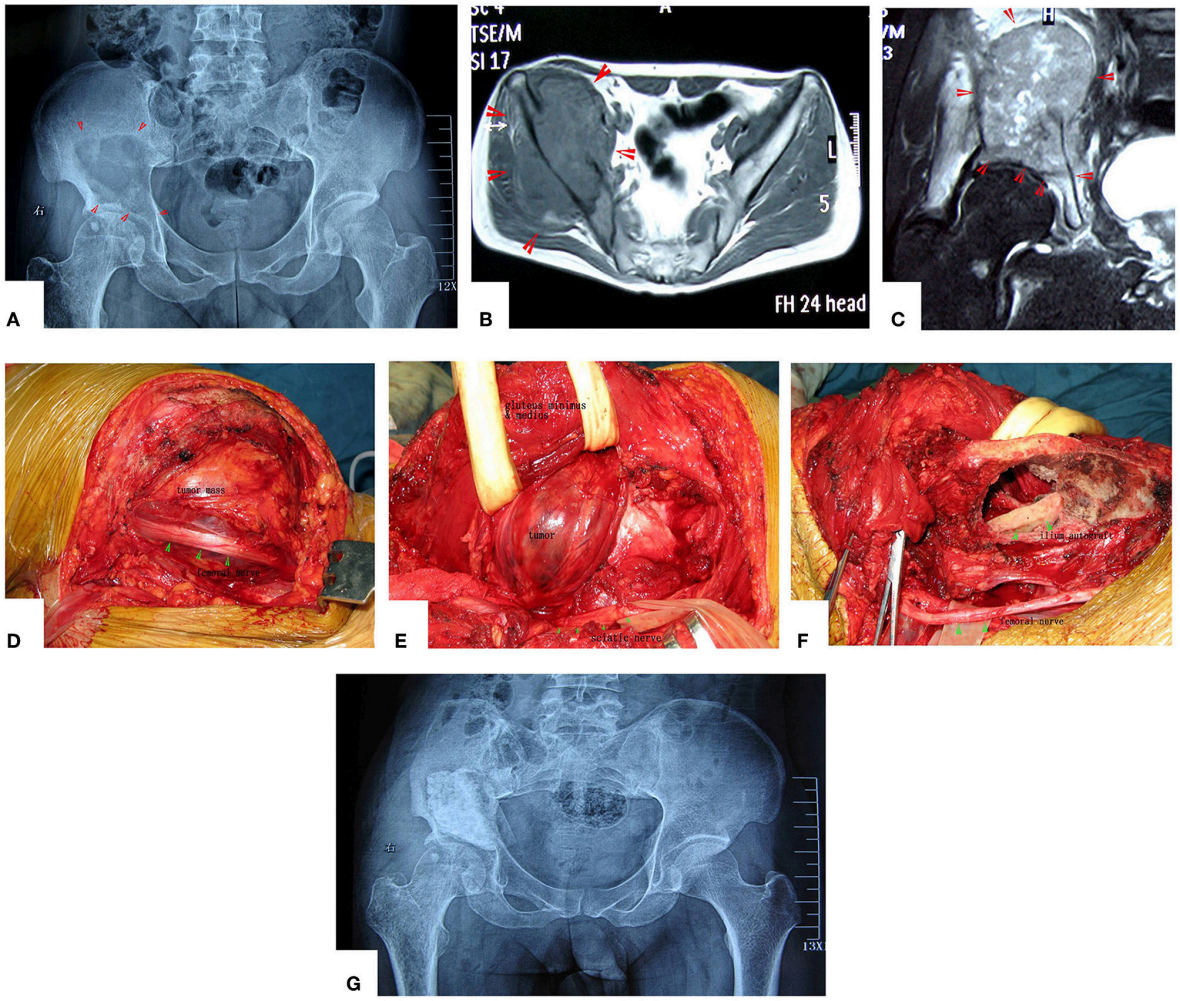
A patient with pelvic MHF treated by MWA. (A) X-ray before surgery shows osteolytic destruction of the cortical bone and extraosseous tumor expansion. (B,C) MRI shows a massive tumor very close to the hip joint. Notably, the hip joint is not infiltrated. (D) Internal pelvic space exposure; notice the compressed femoral nerve. (E) The loose, devitalized tumor tissues were removed after MWA. (F) The re-strengthening procedure included using an autograft from the ilium and a mixture of cement and morselized allograft bone chips. (G) X-ray after the operation.
